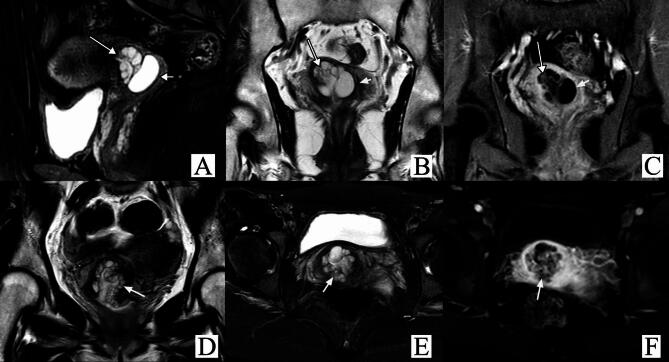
MRI of 2 PJS patients with LEGH. A–C: A 38-year-old patient was classified as Grade 2. She was pathologically confirmed to be LEGH. Sagittal (A) and coronal (B) T2WI showed dense microcysts (long arrow) and macrocysts (short arrow) in the cervical canal. The cervical stromal ring was intact with a low signal density on T2WI (B), and the proportion of microcysts was less than 1/3. Coronal contrast-enhanced T1WI (C) showed that the microcysts were mildly enhanced. D–F: A 27-year-old patient was classified as Grade 3. She was pathologically confirmed to have LEGH with increased vaginal discharge for more than 1 year. Coronal (D) and axial (E) T2WI showed dense microcysts and macrocysts in the cervical canal (arrow). The cervical stromal ring was intact with a low signal density on T2WI (E), and the proportion of microcysts was more than 1/3 but less than 2/3. Axial contrast-enhanced T1WI (F) showed that the microcysts were moderately enhanced.
